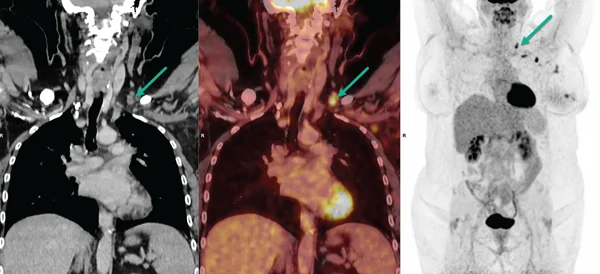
Coronal CT and fused 18F‐FDG PET/CT images and maximum intensity projection coronal images revealing increased FDG uptake in the supraclavicular lymph node metastasis. In addition, increased tracer uptake in the carcinoma in the left breast and the axillary lymph node metastases.
